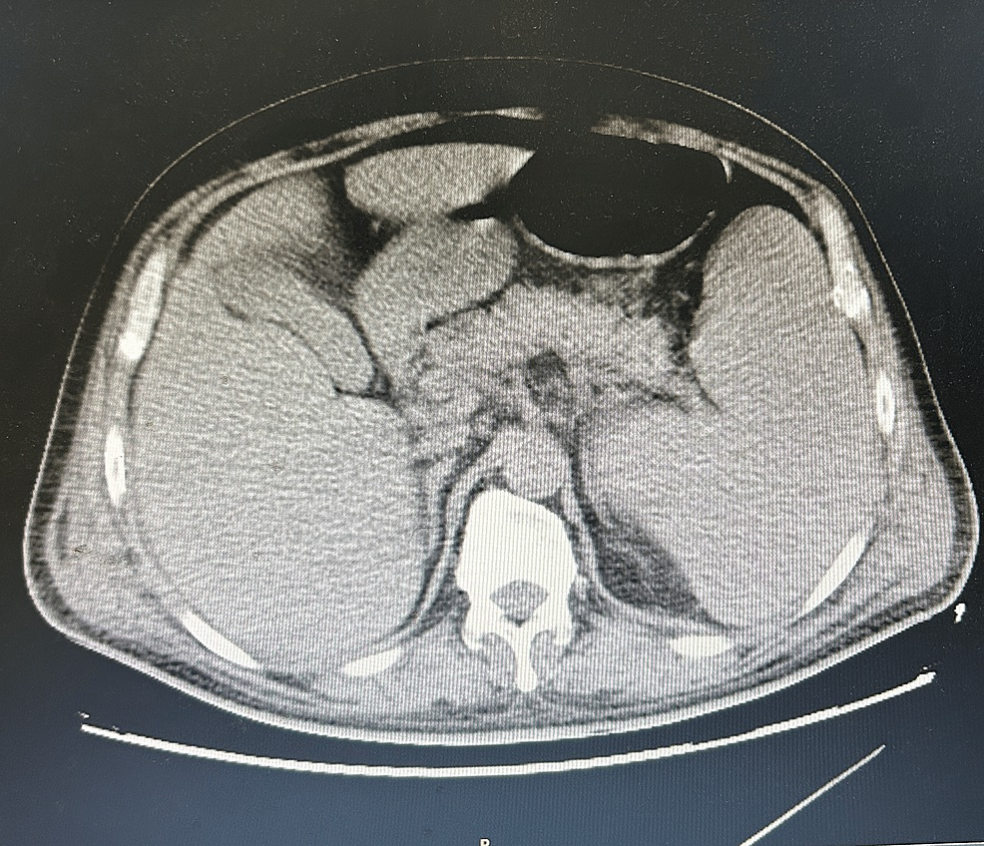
Abdominal CT An abdominal CT scan revealed a substantial increase in spleen size.
